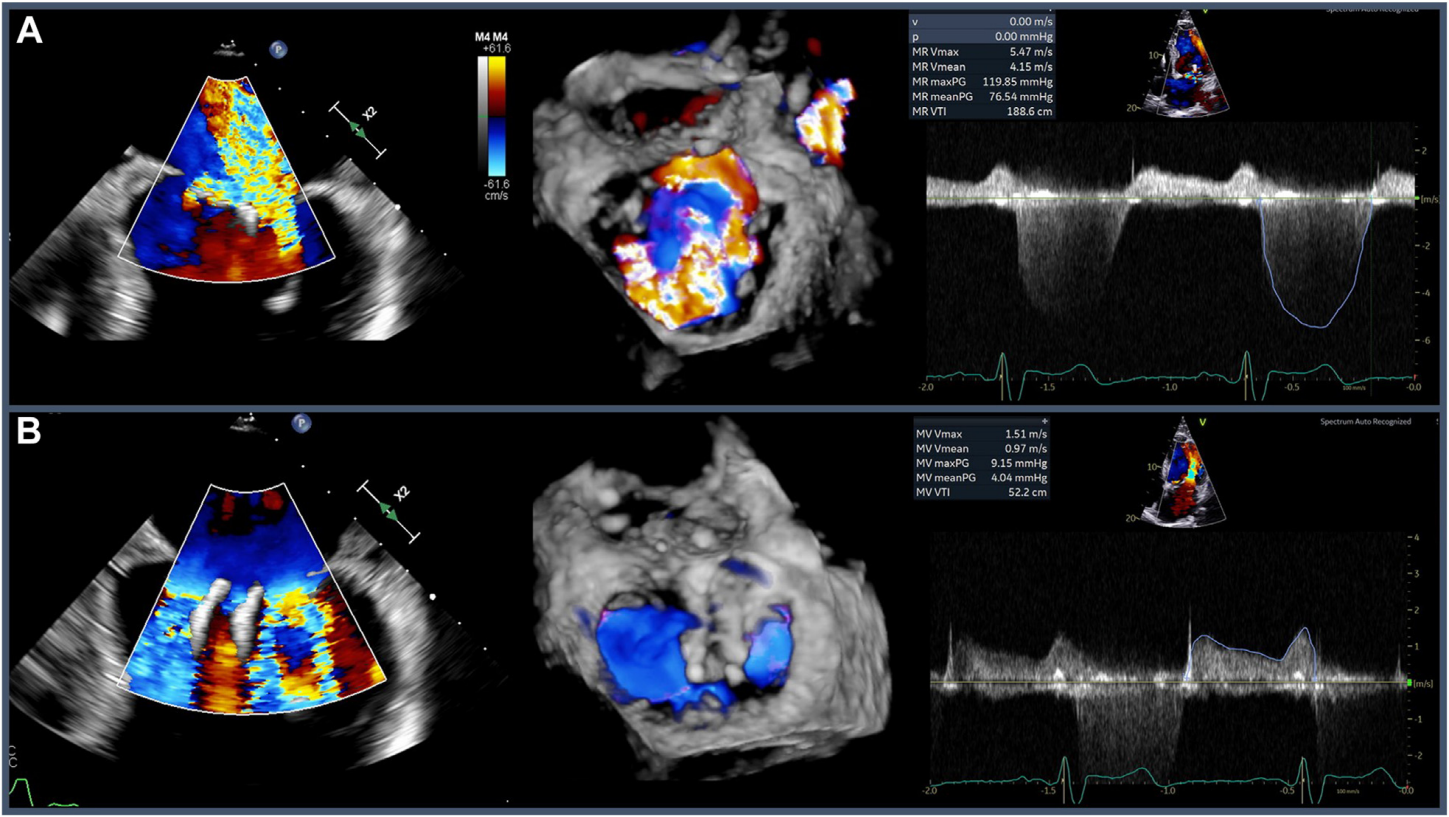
Pre-procedure transesophageal echocardiogram. (A) Transesophageal echocardiogram bicommissural view at 60 degrees of the mitral valve with color Doppler demonstrating severe mitral regurgitation (MR) with both lateral and medial jets. Accompanied by 3D reconstruction and transthoracic echocardiogram pulse wave Doppler demonstrating holosystolic regurgitation with a maximum velocity of 5.47 m/s. (B) Transesophageal echocardiogram bicommissural view at 60 degrees of the mitral valve with color Doppler demonstrating turbulent flow across the valve in the setting of mitral stenosis. Accompanied by 3D reconstruction and pulse wave Doppler measuring peak gradient of 9 mm Hg and a mean gradient of 4 mm Hg.
